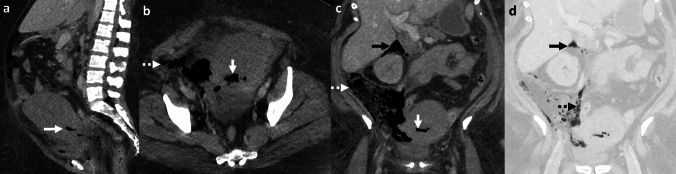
Gas gangrene of uterus. A 35-year-old with intrauterine fetal demise delivered vaginally with chorioamnionitis and puerperal sepsis. CT images show gas in the lower uterine segment (solid white arrows, a–c), suggesting gas gangrene of the uterus. Gas further extends into the right extraperitoneal pelvis (dotted white arrows, b, c, and dotted black arrow, d) and into the right retroperitoneum (solid black arrows, c, d), suggesting secondary retroperitoneal fasciitis. The patient underwent hysterectomy and abdominal washout
